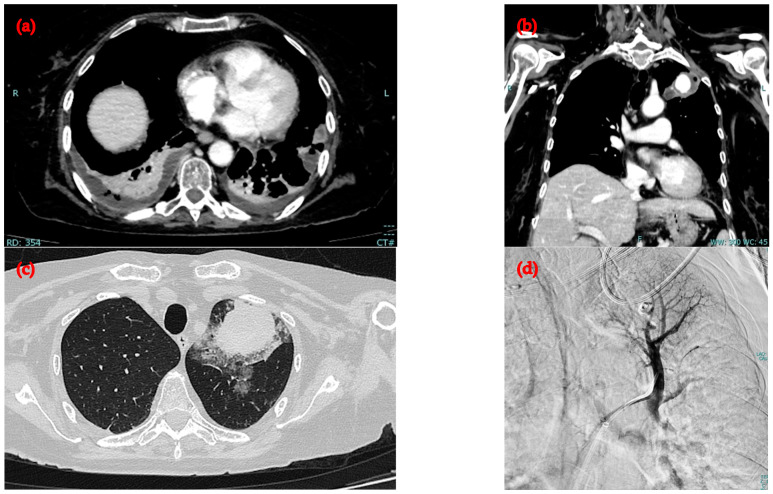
The CT images with contrast show the following: (a) a basal right pleural effusion with consolidation and multiple air bubbles within; (b) in the apico-dorsal segment of the left upper lobe, an inflammatory lesion containing an intralesional pseudoaneurysm of the apico-dorsal segmental branch of the left upper lobe bronchial artery (maximum diameter 2.3 cm); (c) alveolar hemorrhagic involvement following the rupture of the pseudoaneurysm; (d) embolization procedure through the placement of 5 coils.
